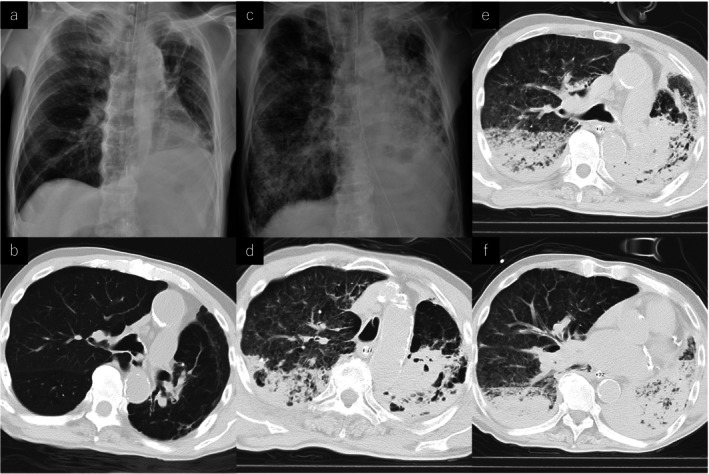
Chest X‐ray and chest computed tomography (CT) scans. (a) Chest X‐ray 30 min after the start of transfusion. (b) Chest CT scan on arrival (before transfusion). (c) Chest X‐ray 9 h after the start of transfusion. (d, f) Chest CT scans obtained at various lung levels 6 h after the start of transfusion.
